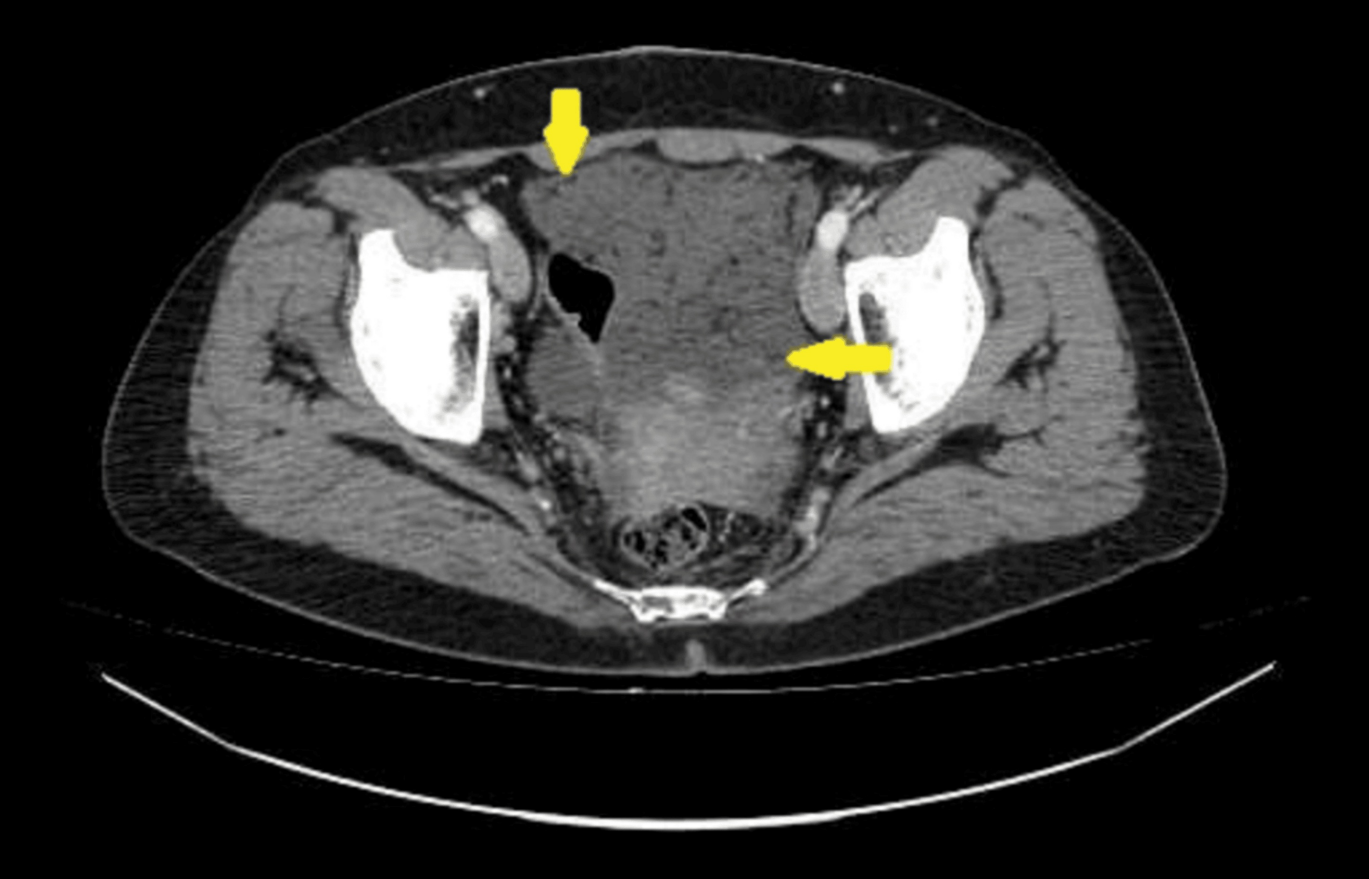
CT imaging of peritoneal metastases and local fluid accumulation in the abdomen and pelvis. Arrows show metastases in the greater omentum.
